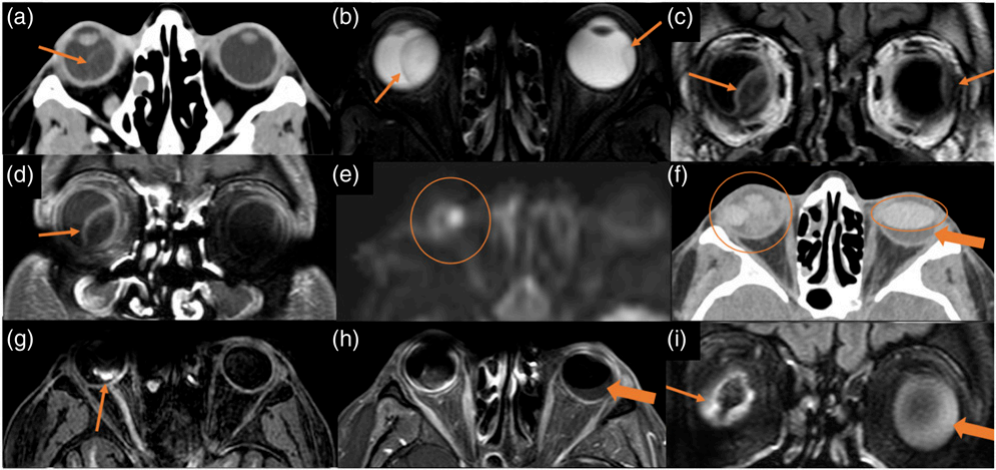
Choroidal abscesses and subretinal haemorrhage. 25-year-old woman with cystic fibrosis underwent double-LT. She had severe retro-ocular headache 4 days post-transplant and both CT and MRI scans were performed. Figures: axial CT scan (a), axial T2-weighted sequence (b), coronal FLAIR sequence (c), axial intravenously enhanced T1-weighted (d) and axial diffusion sequence (e). There are subretinal collections in both eyes (arrows in a–d), showing internal hyperintensity on T2 and FLAIR, with restricted diffusion, and peripheral enhancement. These findings are consistent with choroidal abscesses. During the follow-up, she underwent surgery with intraocular silicone placement. Figures: axial CT scan (f), non-contrast 3DT1 sequence (g), axial T1-weighted sequence (h) and coronal FLAIR sequence (i). Posterior to the left intraocular silicone, there is a crescent-shaped collection, hypodense on CT scan, hypointense on T1 and hyperintense on FLAIR, compatible with subretinal haemorrhage. There is poor visualization of the right ocular globe due to magnetic susceptibility artefact. The left intraocular silicone is irregular and has moved anteriorly. There is posterior right chamber occupation with high-density areas on the CT scan and hyperintense areas on T1, without evidence of enhancement, compatible with subretinal haemorrhages.
